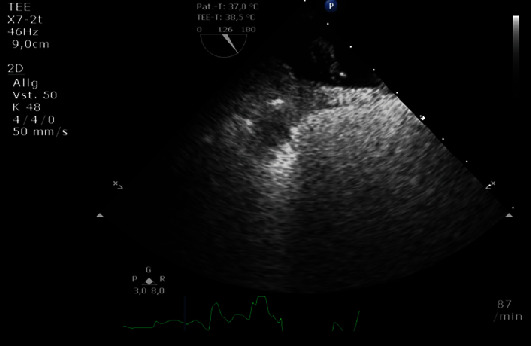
Transoesophageal echocardiography (TOE) demonstrating a large PFO with right-to-left shunt after bubble contrast injection.
